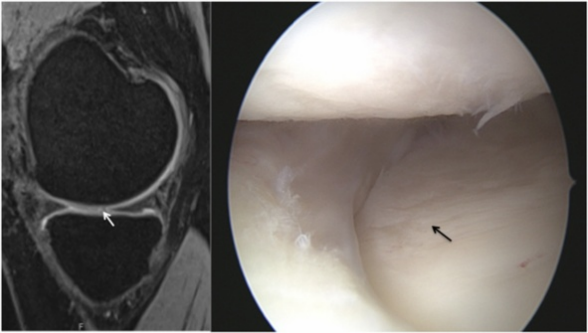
MRI and arthroscopic image of a grade II lesion in a 32-year-old male patient, reported by both readers. It is well visible in the sagittal images
